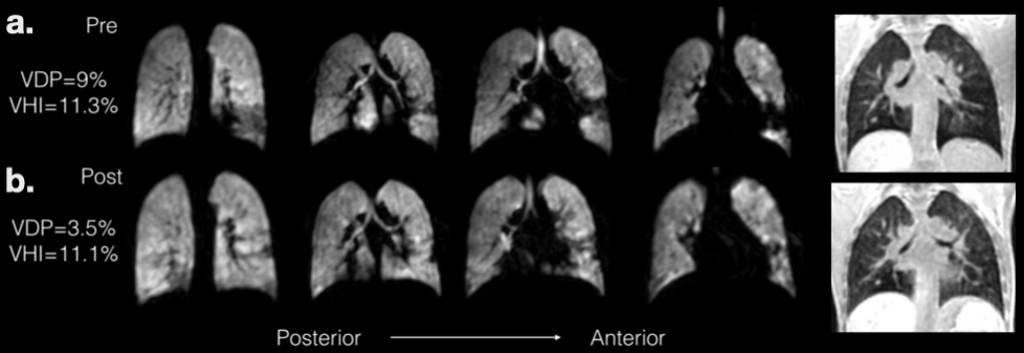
Patient A (13 years): clinical diagnosis of PCD; referred due to questionable clinical benefit of their standard physiotherapy. At baseline, spirometry showed a mixed obstructive/restrictive defect, suggesting an obstructive pattern with a significant gas-trapping component. Pre-physiotherapy ^129^Xe ventilation images (a) show clear evidence of ventilation defects in the lung bases at baseline. Physiotherapy was performed using a PEP device. Post-physiotherapy ^129^Xe MRI (b) exhibited a marked reduction in the degree of ventilation defects, although some abnormalities remained. Whilst VDP decreased from 9.0 to 3.5%, there was no clear change in VH_I_. Post-physiotherapy spirometry showed an increase in FEV_1_ of 8.4%, but no change in FVC. Complementary ^1^H SPGR imaging at RV (far right panel) indicated a visible decrease in gas trapping in the locations where ventilation defects improved, though some remaining gas trapping was evident. Complementary structure-function (^1^H-^129^Xe) MRI helped assess the benefits of performing the physiotherapy regime in this patient. Despite small improvements in FEV_1_, a clear improvement in regional ventilation and a significant reduction in VDP was observed. FVC, forced vital capacity; PEP, positive expiratory pressure; VDP, ventilation defect percentage.
